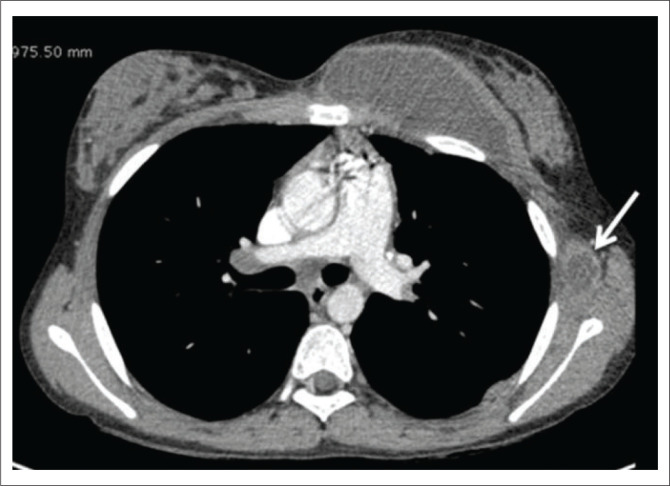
A 14-year-old female presenting with a left breast swelling. Axial post-contrast computed tomography image shows a large chest wall abscess involving the left pectoralis muscle with involvement of the costal cartilage and 5th rib. A similar but smaller collection was seen in the left subscapularis muscle (white arrow).
